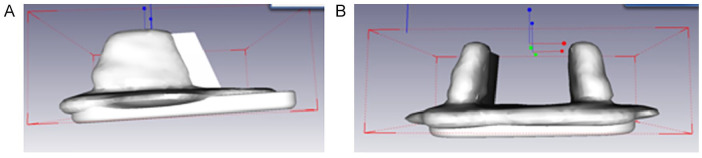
Assessment of prosthesis placement based on computed tomography scans: A. Sagittal plane. B. Frontal plane. A coordinate was applied to the planned prosthesis component and to the implanted prosthesis component. Thereby, the translational and angular deviations are evaluated.
